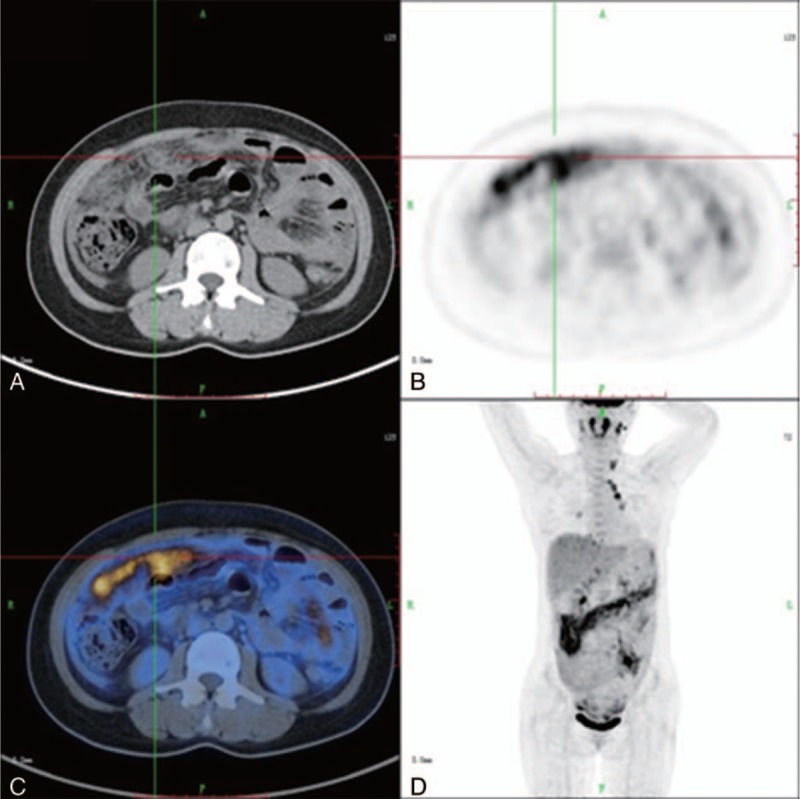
PET/CT images of a false-positive case, a 41-year-old woman who presented with peritoneal thickening: axial CT (A), axial PET (B), axial fused PET/CT (C), and 3D PET (D). The patient had abdominal pain and distention for 3 weeks. She had serum CA12-5 of 1126 U/mL, normal serum α-fetoprotein, normal carcinoembryonic antigen, and normal CA19-9. PET/CT shows increased ^18^F-FDG uptake in the peritoneum (SUV_max_ of 5.6), mimicking a malignant lesion. Peritoneal biopsy confirmed that the patient had peritoneal tuberculosis. CT = computed tomography, FDG = fluorodeoxyglucose, PET = positron emission tomography, SUV_max_ = maximal standardized uptake value.
